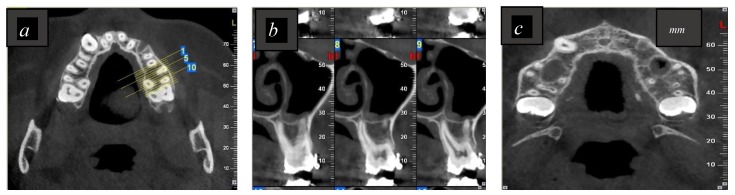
Representative cone beam computed tomography (CBCT) images showing the palatal root apex not in contact with the sinus floor (MSF). (a,c) A maxillary axial CBCT view; (b) a maxillary sagittal CBCT view.1; 5 and 10 are the slices numbers.
